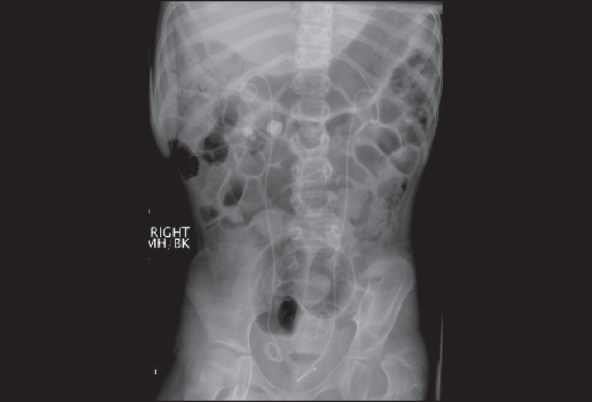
Plain film KUB. Male child with profound developmental disabilities. Patient presented with bilateral obstructing renal calculi. Patient underwent placement of bilateral ureteral stents and staged extracorporeal shockwave lithotripsy. KUB obtained to evaluate response to therapy. KUB demonstrates bilateral residual stone fragmentsEvaluate the structure of the spineEvaluate retroperitoneal air and fat-fascial planes (abscess, infection, perforation)Detect air in the GU tract or free air in the abdomenImpacted feces (constipation)Calcifications (urolithiasis)Detect retained foreign bodiesEvaluate the position of stents or drainsPlain film X-ray of the pelvisTo evaluate the bony pelvis and the terminal spineEvaluate for any calcifications or foreign body within/adjacent to the urinary bladder or urethra
